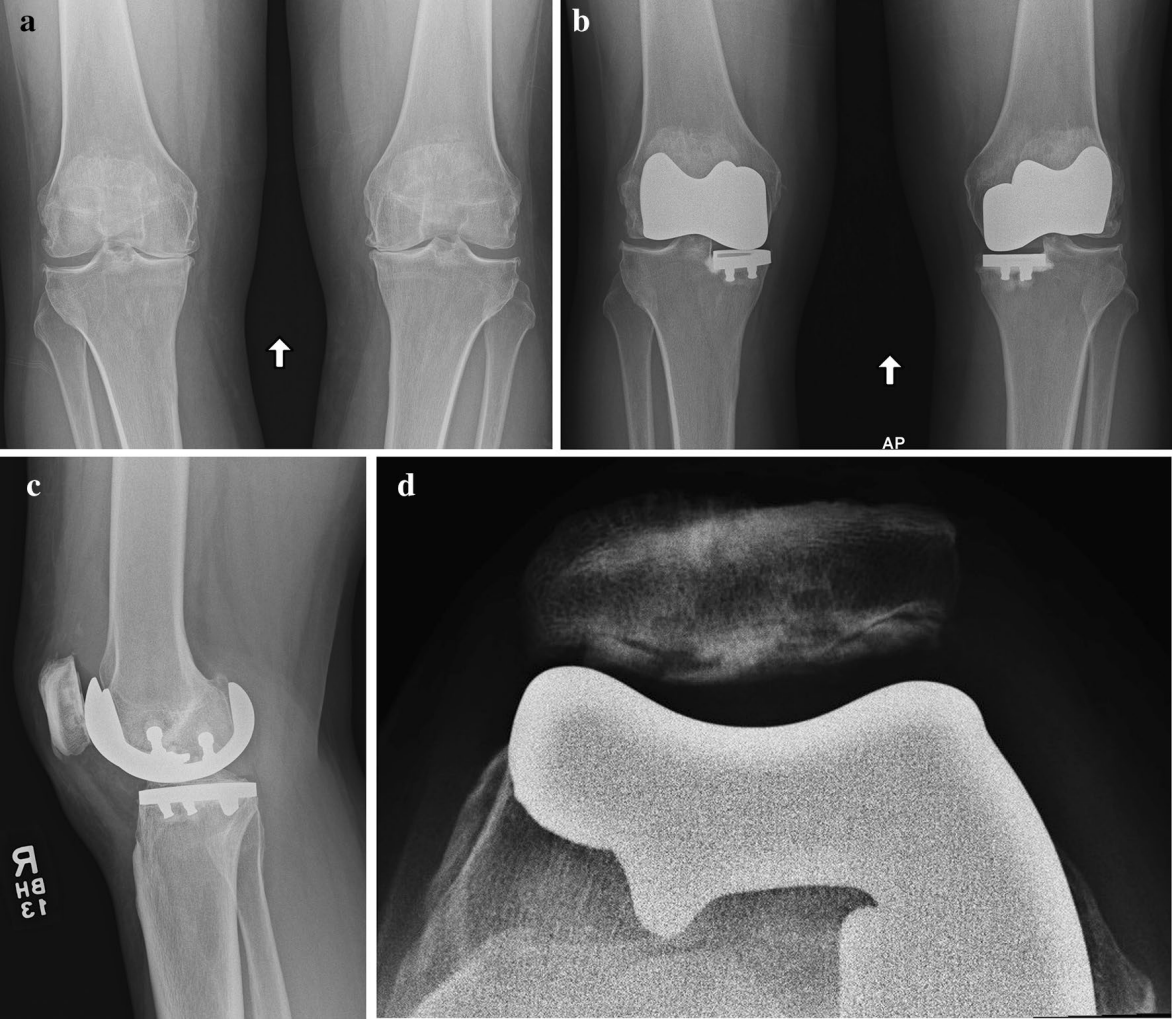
Bicompartmental knee arthroplasty (BKA). Pre-operative bilateral anteroposterior radiograph of a 44-year-old woman with disabling anterior and medial joint pain (a). Post-operative bilateral anteroposterior (b), lateral (c), and skyline (d) radiographs of a patient after bilateral BKA combined medial and patellofemoral replacement
